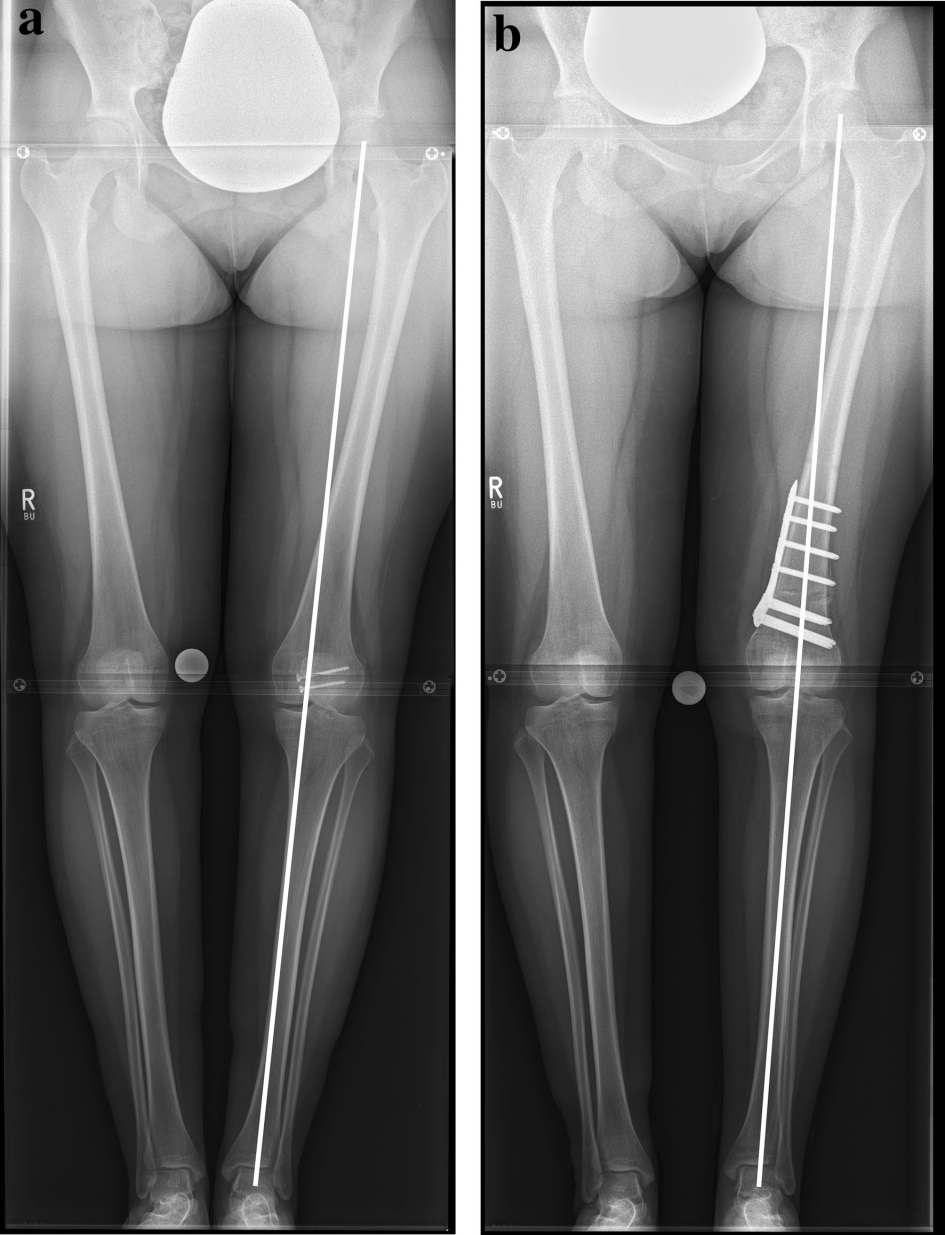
a Preoperative and b postoperative standing radiographs of a 20-year-old female patient. Isolated supracondylar external rotation osteotomy of the lift femur was performed with reduction of the femoral antetorsion from 30.0° to 15.8°. By this, the mLDFA decreased from 92.9° to 91.5° and the ischiofemoral space increased from 12 to 16 mm. Two screws were removed from the patella after an ipsilateral patella fracture in the past
